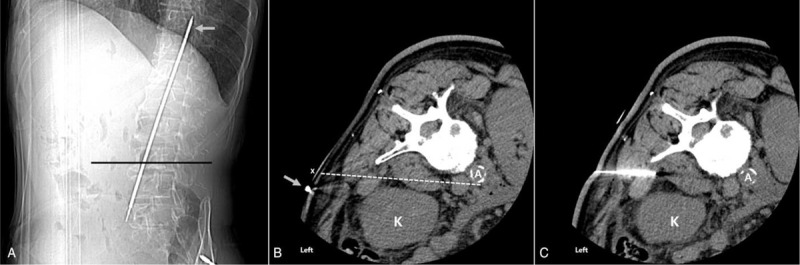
(A) Computed tomography (CT) scout view. (B) CT fluoroscopic image showing the skin entry point (X) and a safest and shortest imaginary line (dotted line) to reach the target point by avoiding the kidney. (C) CT fluoroscopic image showing the Chiba needle. Metal wire (gray arrow) and A (aorta).
